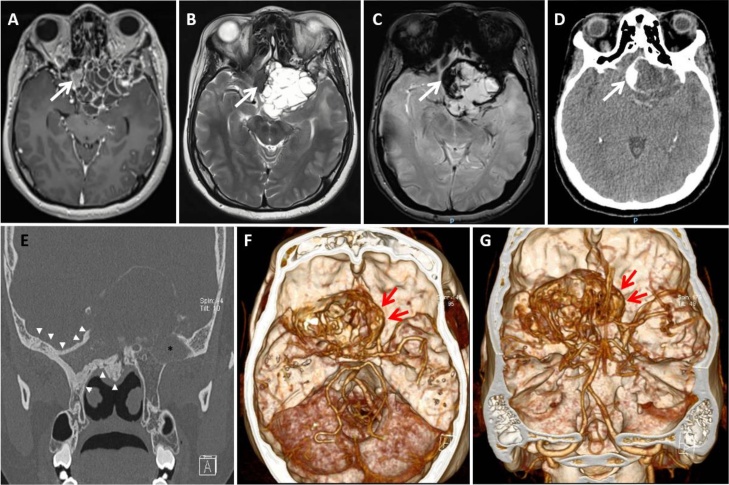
Preoperative Brain Magnetic Resonance-Imaging (MRI) and Computed tomography-scan (CT-scan). Axials (A) T1 + Gadolinium, (B) T2-weighted, (C) Gradient echo sequence with a large poli-cystic enhancing selar lesion with extensive osteophytic reaction and invasion of the ipsilateral orbital apex associated with fibrous dysplasia and an enhancing selar mass lesion with their respectively Calcium intensities (*white arrows*). CT-scans (D) axial CT-scan showing the calcified pituitary gland (*white arrow*) and (E) coronal on bone density revealing thickness of the diploe on the right sphenoid wing (*white arrow heads*) and osteolytic lesion on the left orbital apex (*black asterisk*). (F and G) axial and coronal 3D bone reconstruction showing calcified pituitary gland (*red arrows*) and a calcified rim around the tumor.
